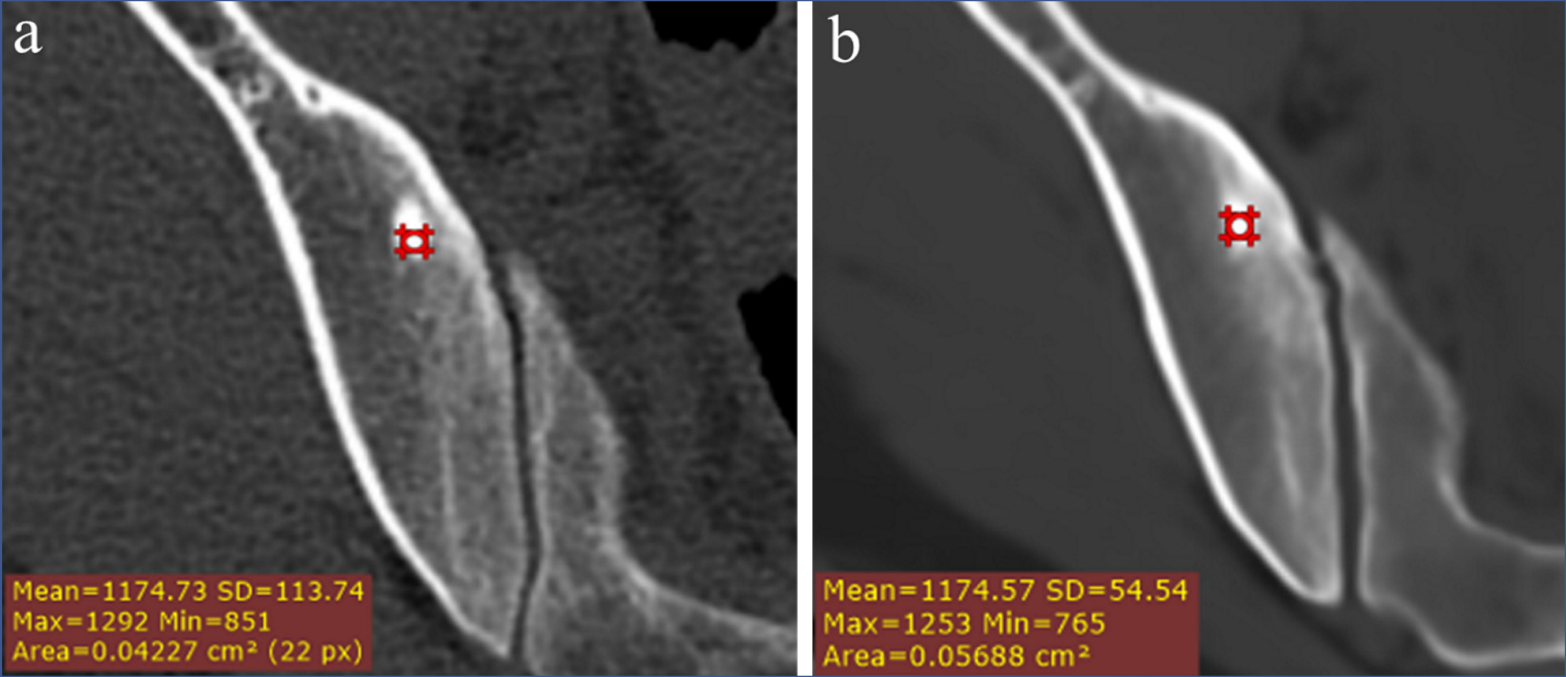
Hounsfield Units maps. An incidental enostoma in the right ilium shows the same amount of Hounsfield Units on conventional CT (a) as the signal intensities on synthetic CT (b). The measurement of Hounsfield Units on synthetic CT can be very useful, for example in the differentiation of sclerotic lesions. A mean attenuation of 885 HU and a maximum attenuation of 1060 HU are often used as cutoff values in clinical practice – when lower than these reliable thresholds a metastatic lesion is the favored diagnosis [38].
